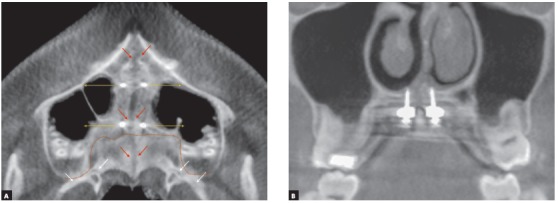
A) CBCT axial slice 15 days after MARPE was completed. Direction of the forces applied to miniscrews (yellow arrows), hypodense area showing opening of midpalatal suture (red arrows) and pterygopalatine suture (white arrows). B) Coronal CBCT slice of posterior MARPE region, showing bicortical anchorage.
